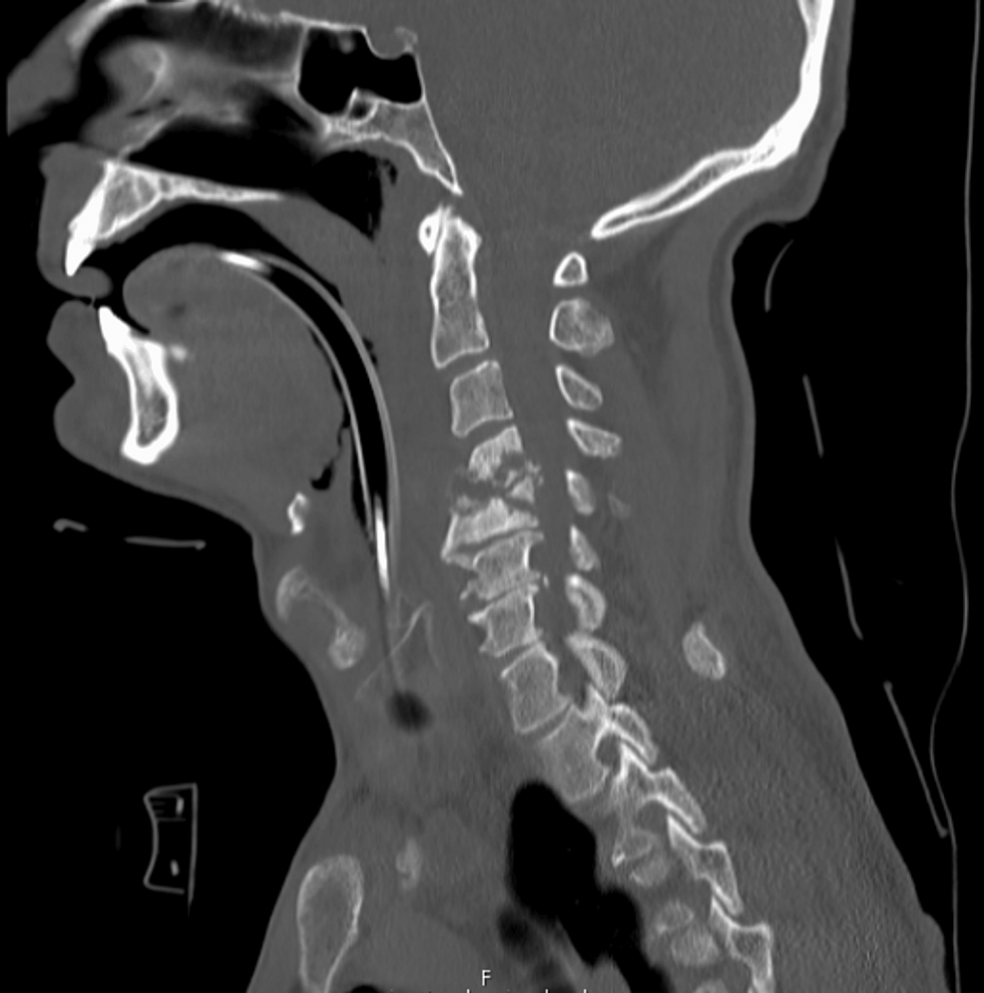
CT neck post drainage of the collection. The prevertebral collection has decreased significantly in size. There is small residual soft tissue thickening with some bone fragments within the prevertebral region. The thin rim of retropharyngeal oedema is seen extending from C2-C5. Bony destruction is seen involving the C4 and C5 vertebral bodies with mild retropulsion which extends into the spinal canal causing canal narrowing, which remained unchanged
